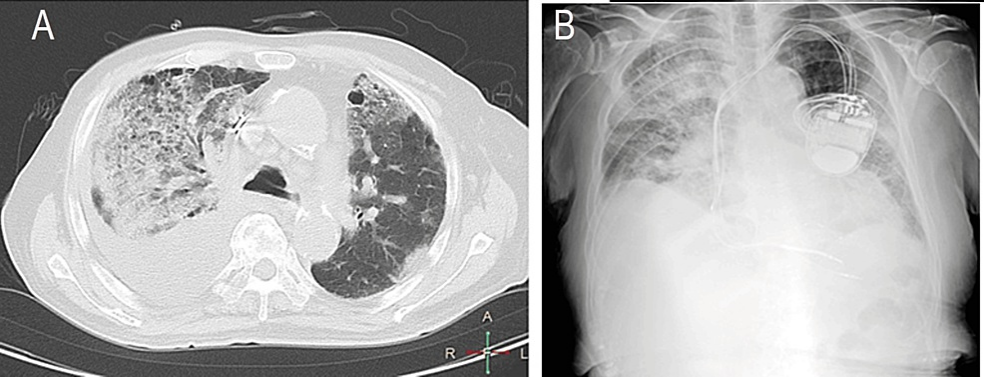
(A) Chest computed tomography on day 1; (B) Chest radiography on day 2
